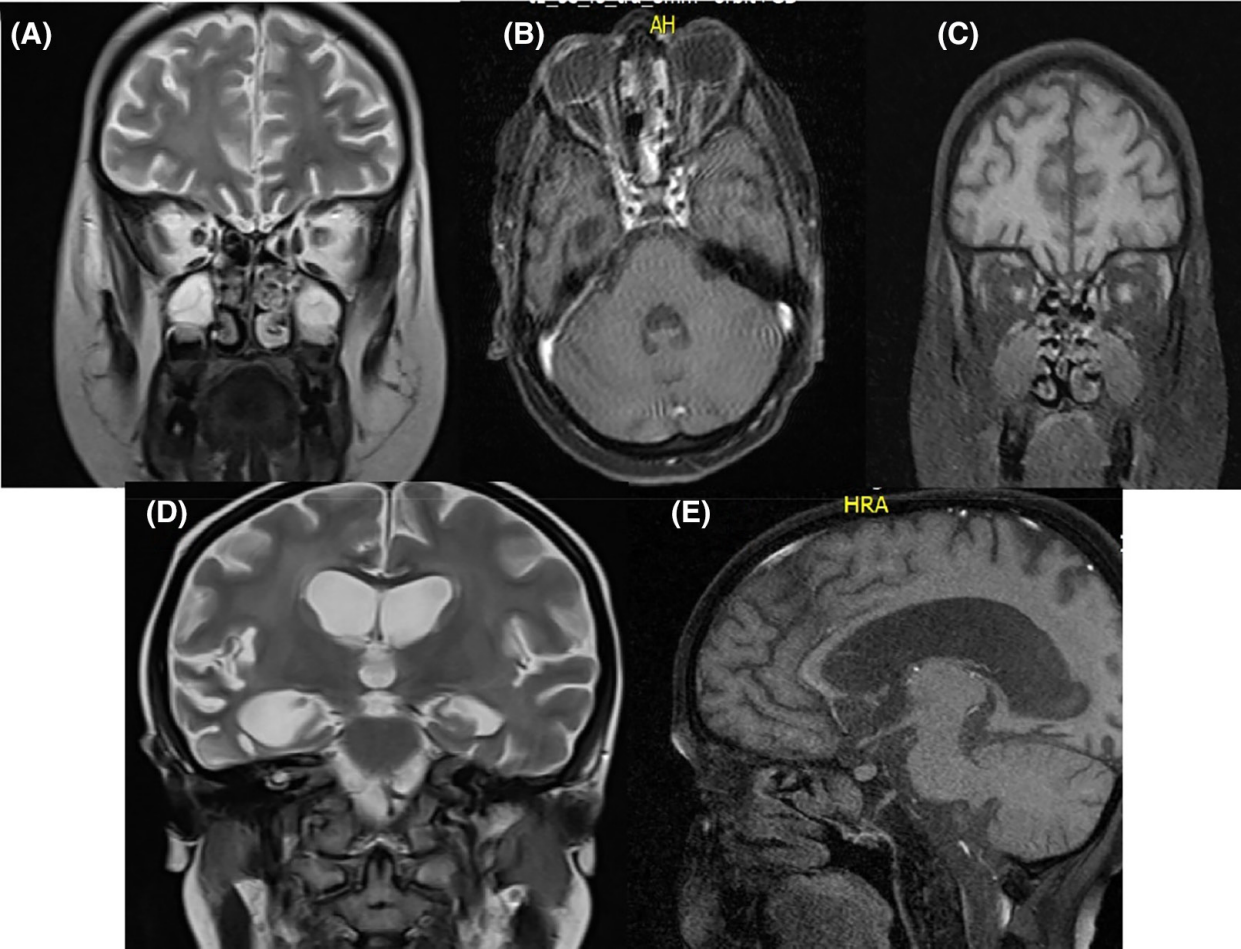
Brain and orbital MRI with and without gadolinium contrast. (A) coronal T2 MRI without contrast shows bilateral optic nerve enlargement (B) axial T1 MRI with contrast shows bilateral optic nerve enlargement and enhancement (C) coronal T1 MRI with contrast shows bilateral optic nerve enlargement and enhancement (D) coronal T2 MRI without contrast shows brain third and lateral ventricles enlargement (E) sagittal T1 MRI with contrast shows brain third ventricle enlargement.
